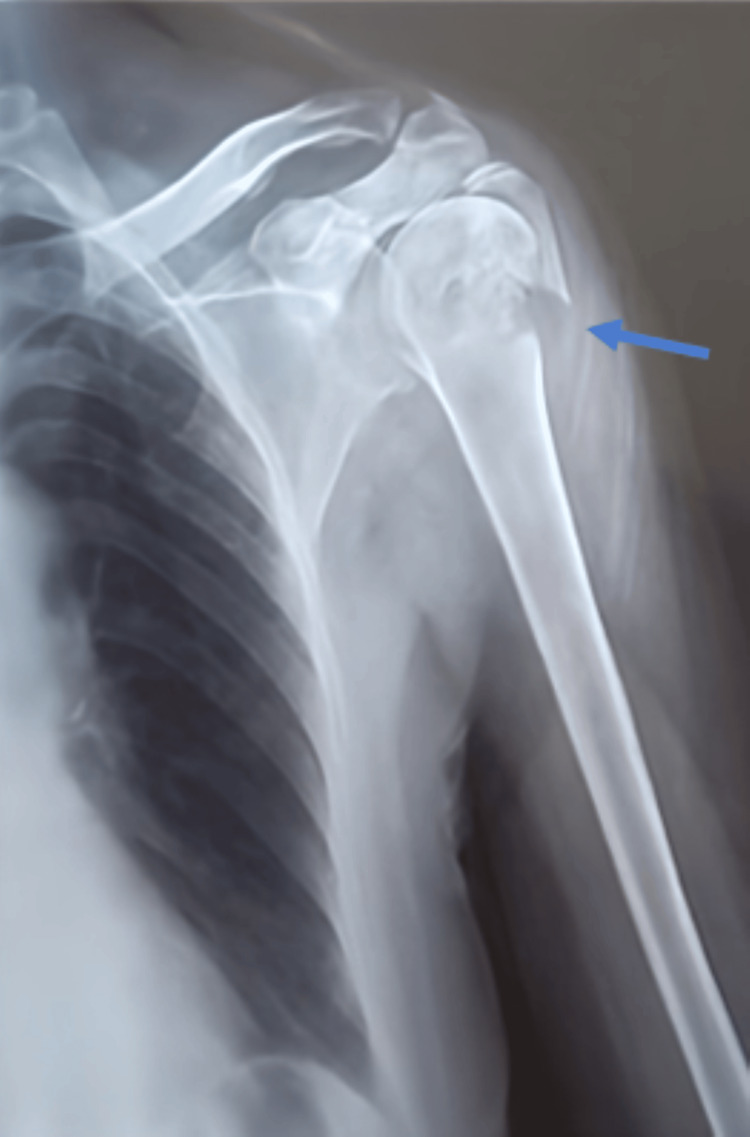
Standard frontal X-ray of the left shoulder showing a complex comminuted fracture-dislocation at the basicervical and humeral head regions with posterior lateral avulsion of a fracture fragment (blue arrow), with infiltration of the adjacent soft tissues
